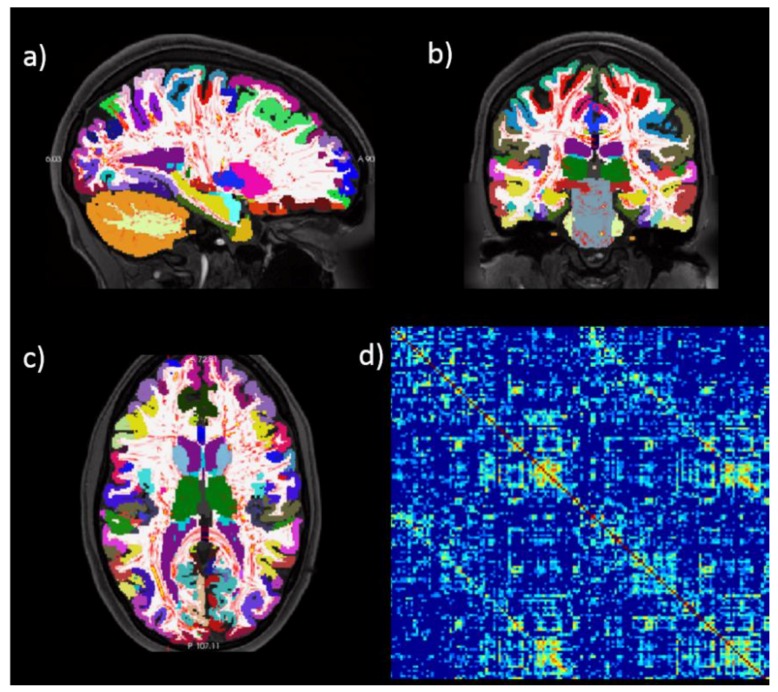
Human connectome estimation. The image shows an example of connectome generation from MR images: (a–c) MR anatomical images can be segmented into several meaningful regions, diffusion imaging provides an estimation of axonal connections linking each pair of parcels (red tracts) for the construction of the structural connectome matrix (d).
